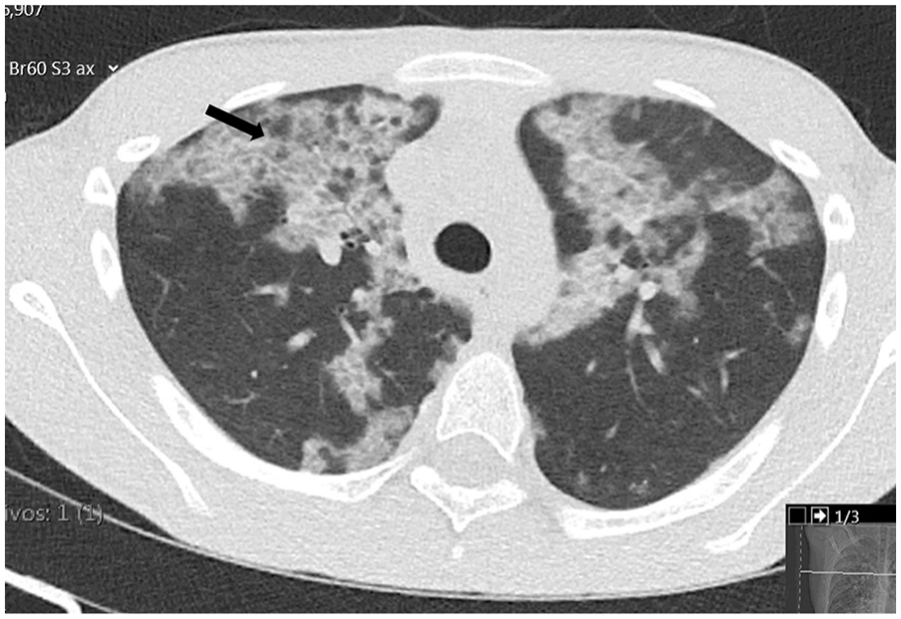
Chest CT showing multiple bilateral areas of ground-glass opacities involving > 65% of lung parenchyma with areas of crazy paving (arrow).
